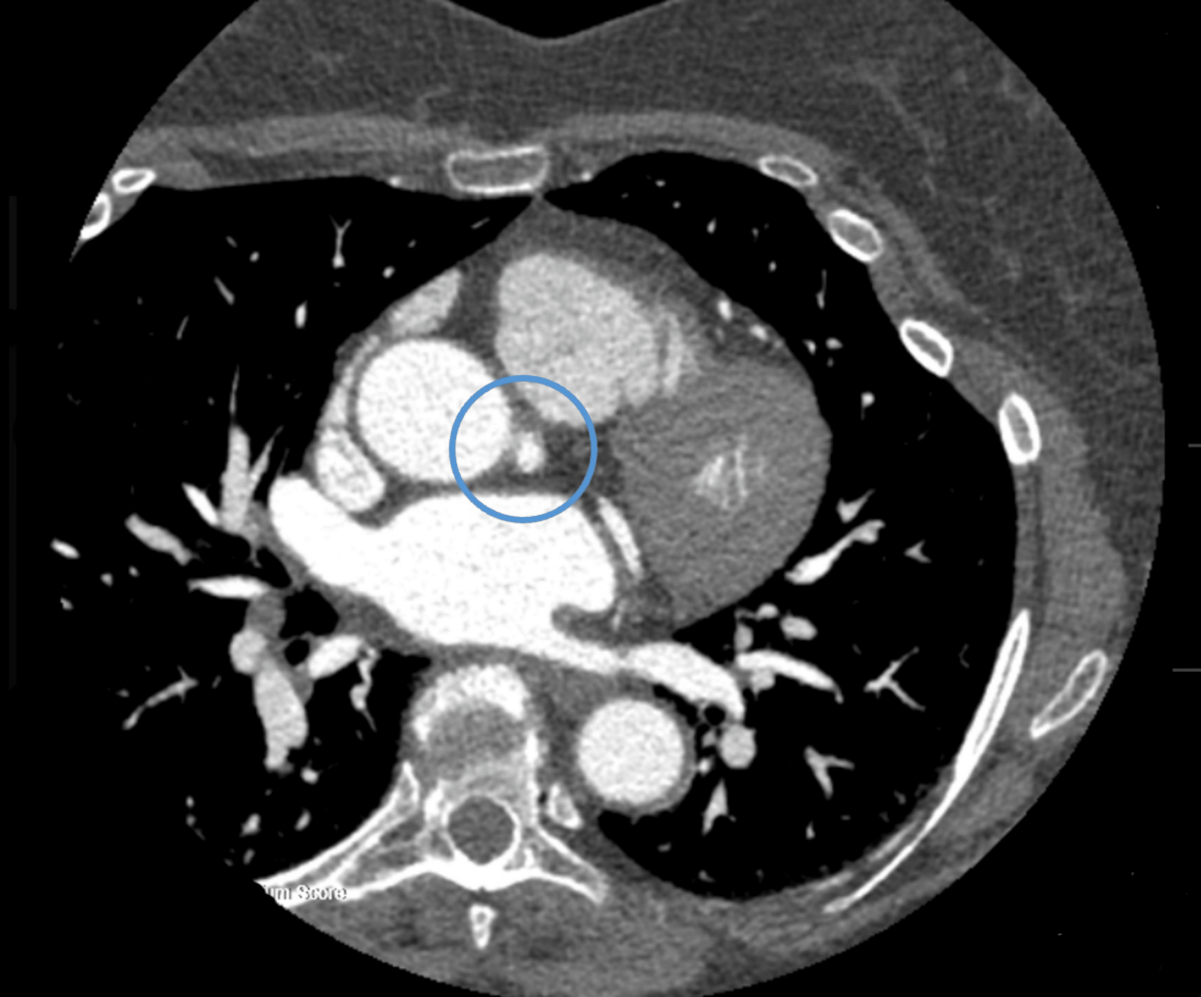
Computed tomography angiography 2D image showing the origin of the left main artery (within the blue circle).
